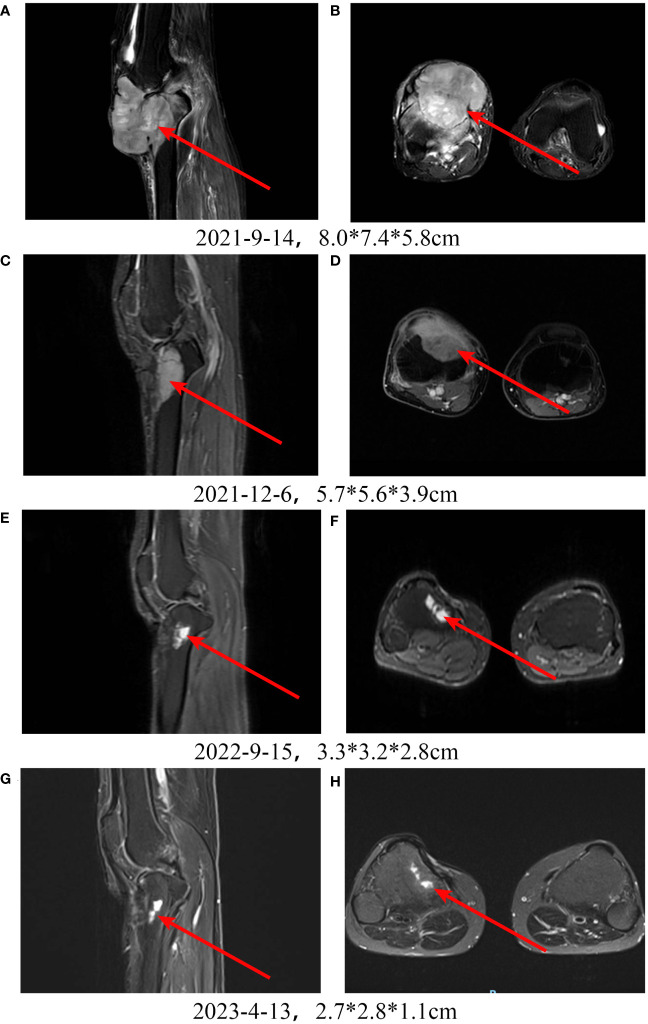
Treatment of a patient with high-grade fibrosarcoma of the right knee with PD-1 inhibitor + chemotherapy + Endostar. A, B: Pretreatment MRI scan shows a large tumor in the right knee. C-H: After receiving PD-1 inhibitor-based combination therapy, the tumor continuously shrinks.
